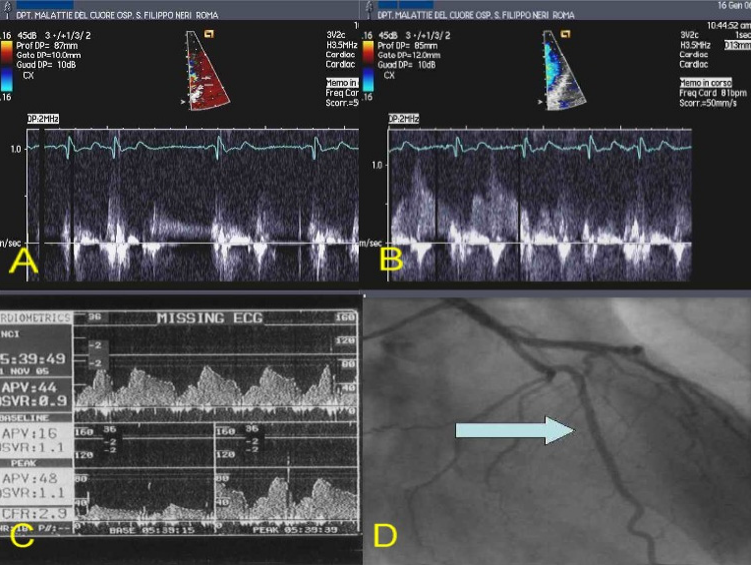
Patients 4. A: TTDE before adenosine. B: TTDE after adenosine. C: DW recording. D: coronary angiography with site of DW recording (arrow). DW: Doppler-wire. TTDE: transthoracic Doppler echocardiography.
